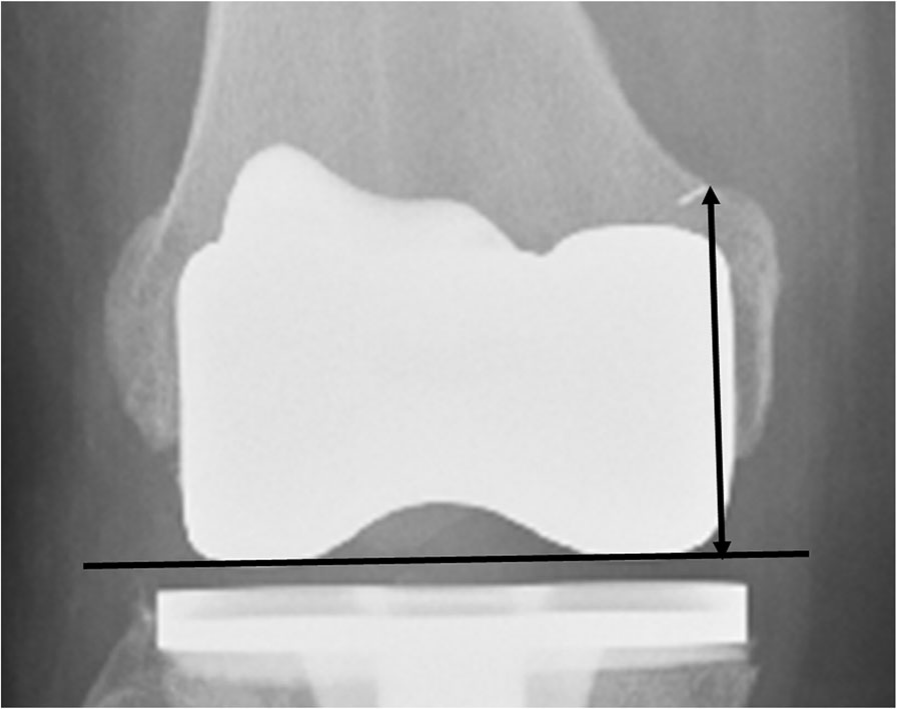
Radiographic joint line distance (RJLD). The distance from the metal marker to the distal condylar surface was defined as the RJLD
